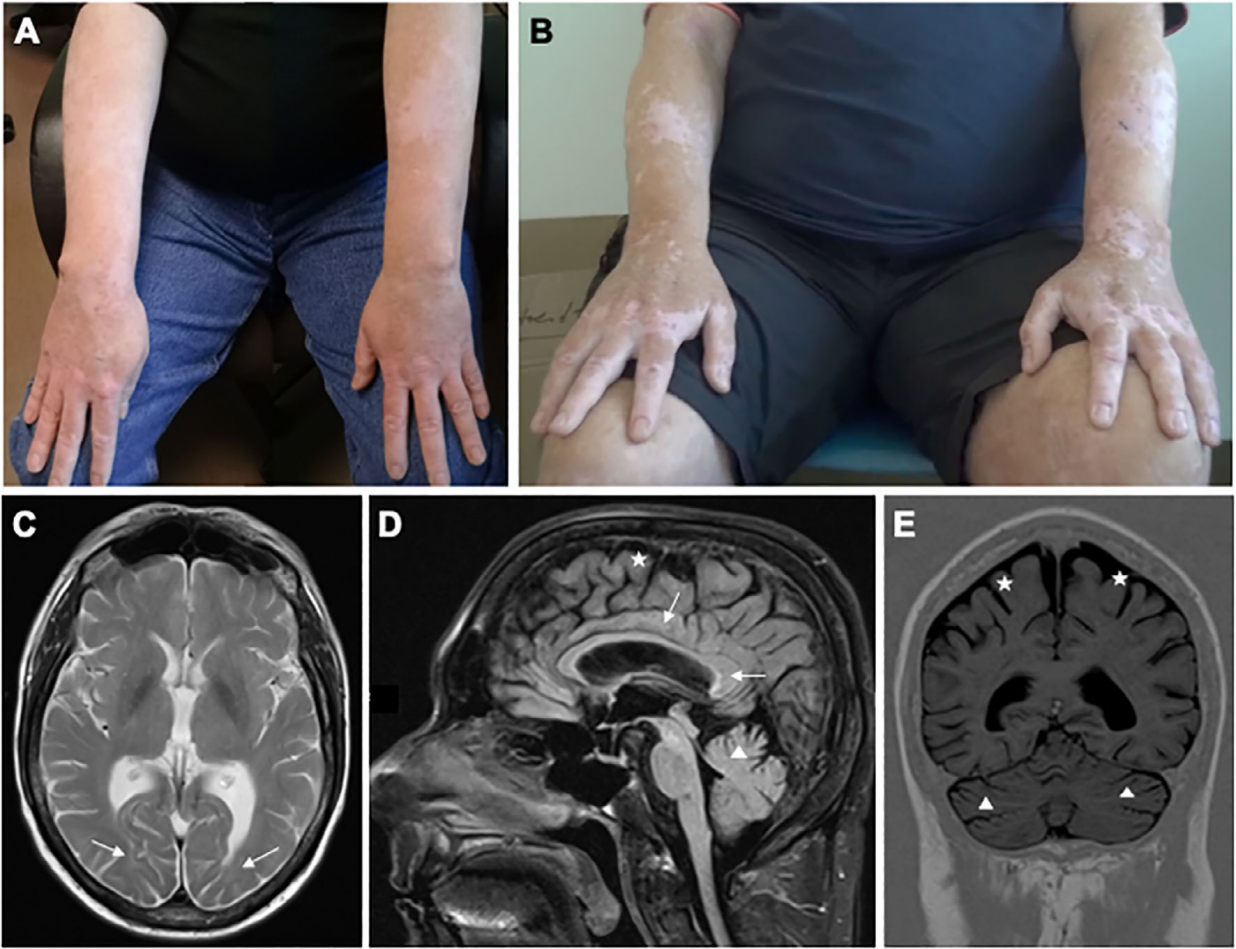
Upper panel: (A) circumscribed, depigmented maculae and patches on the upper extremity. (B) Skin with remarkable hyperpigmentation after short‐time sun exposure. The circumscribed depigmented areas are more pronounced, and some of them show erythema. Lower panel: magnetic resonance imaging at the time of presentation. Mild leukoencephalopathy is noted (C, T2‐weighted axial image, D, fluid attenuated inversion recovery sagittal image, see arrows). Mild generalized brain atrophy is seen (D, E, indicated for the cerebellum [△] and supratentorial brain structures [★]).
